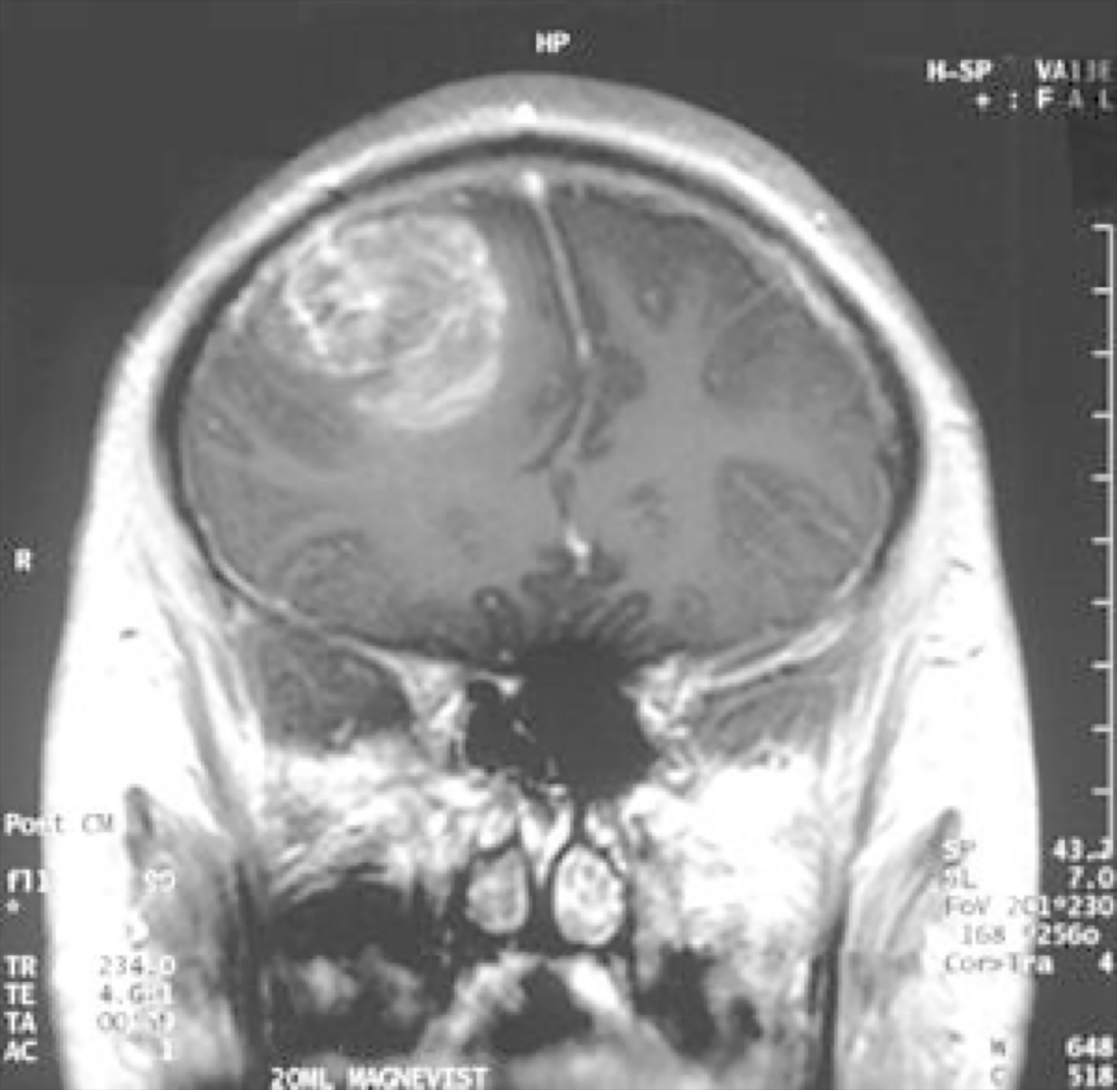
Magnetic resonance imaging (MRI) appearance of glioblastoma: contrast enhancing rind of tumor surrounding a necrotic core (56).
